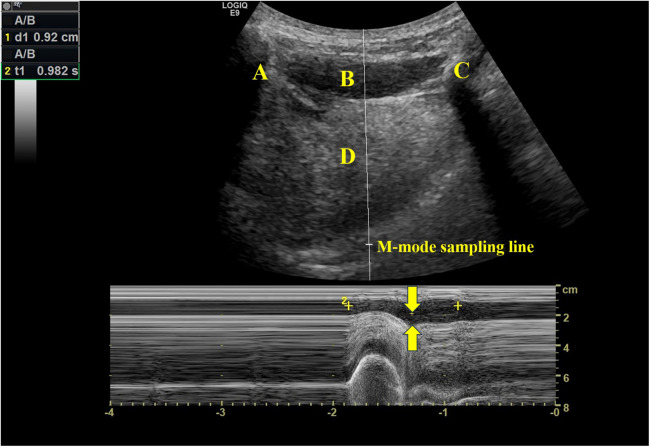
Ultrasound image with four labeled regions A, B, C, and D, and a vertical M-mode sampling line. The lower panel displays an M-mode tracing with yellow arrows indicating measured distances and motion patterns.
